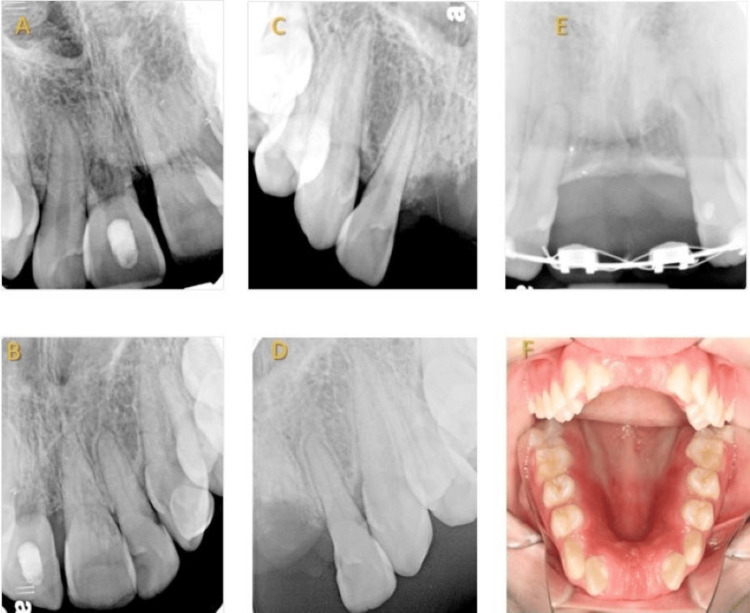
Decoronation of two adjacent maxillary central incisors A 10-year-old healthy girl presented two years after avulsion of the right maxillary central incisor and palatal luxation of the left central incisor. Ankylosis was demonstrated in both central incisors. (A) Preoperative radiograph demonstrating replacement resorption in the right central incisor. (B) External cervical resorption is observed on the distal aspect of the left central incisor. (C) Immediate post-operative radiograph after the decoronation of the right central incisor. (D) Immediate post-operative radiograph after the decoronation of the left central incisor. (E) A six-year follow-up demonstrating ridge augmentation in the horizontal aspect of the ridge. Similar results are observed in both teeth. ECR can be noticed in the distal aspect of the left maxillary lateral incisor. (F) A photograph of the anterior region demonstrating the full width of the ridge after the decoronation six years after the procedure.
